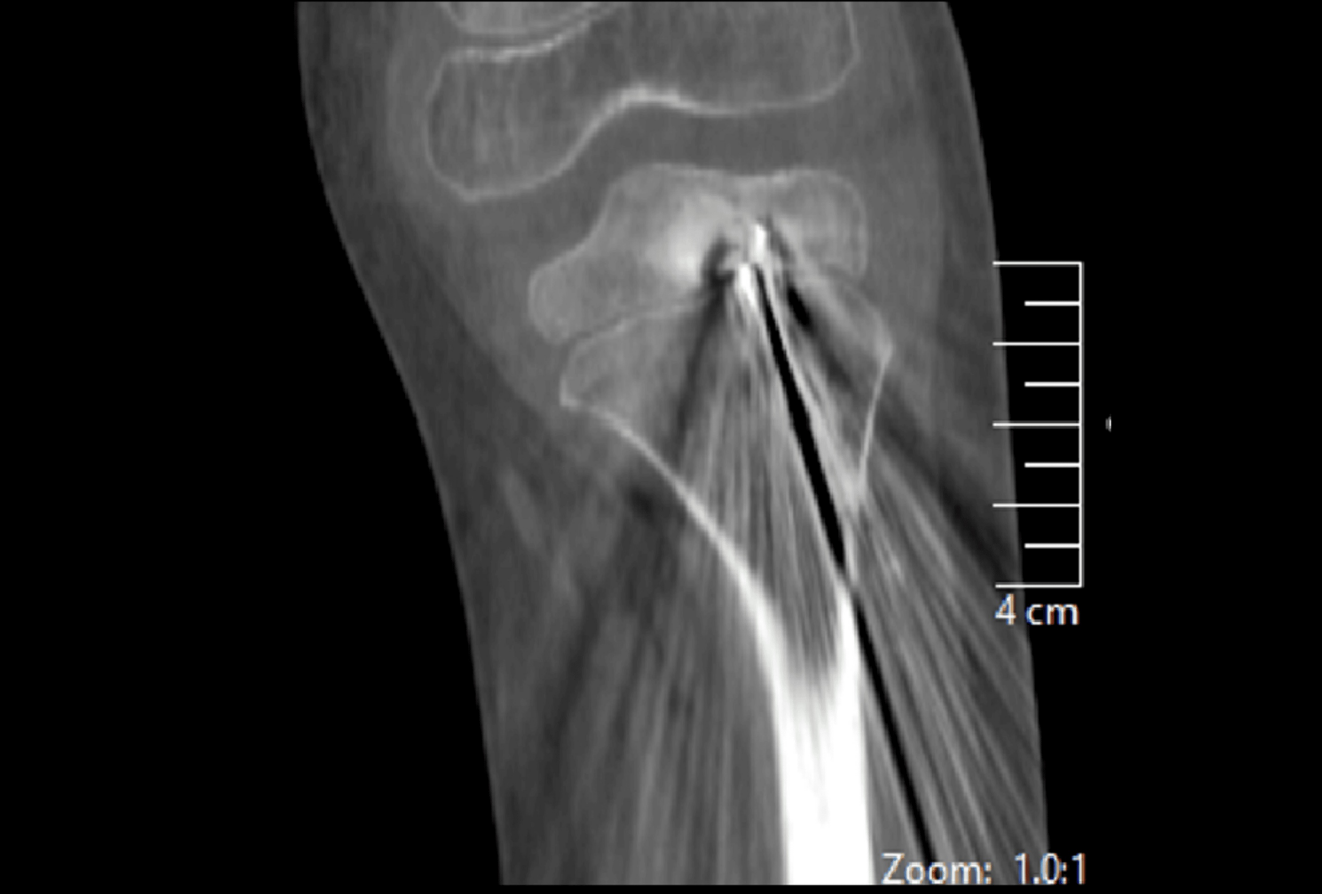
Intraoperative CT coronal image showing the guide wire tip centered in the lesion CT: computed tomography
